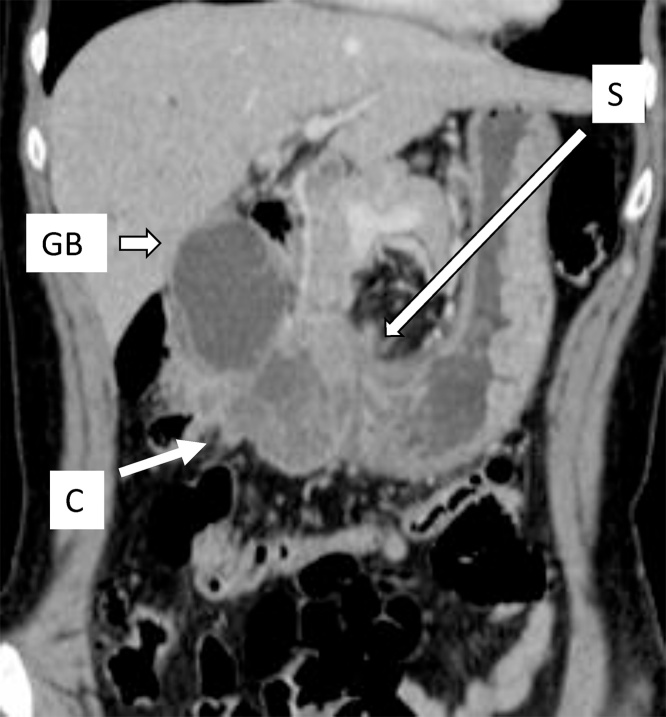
Enhanced CT taken 7 years prior showed the gall bladder (GB) carcinoma (arrow) involving the stomach (S) and right side transverse colon (C), which was resected en bloc.
